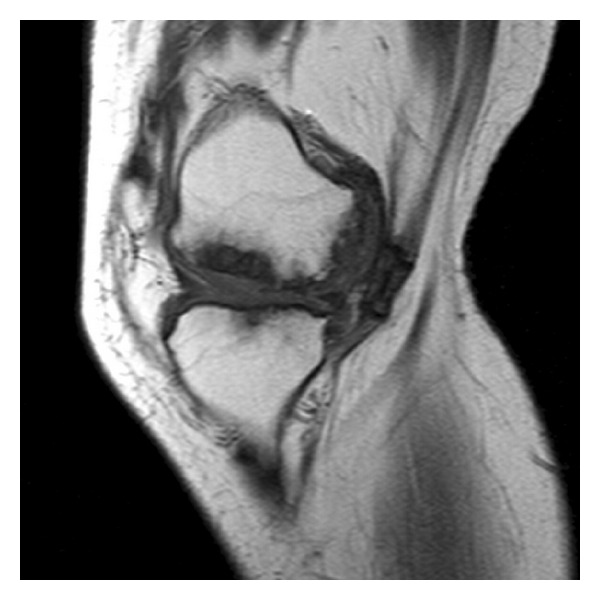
MRI T1 sagittal view of the right knee.
